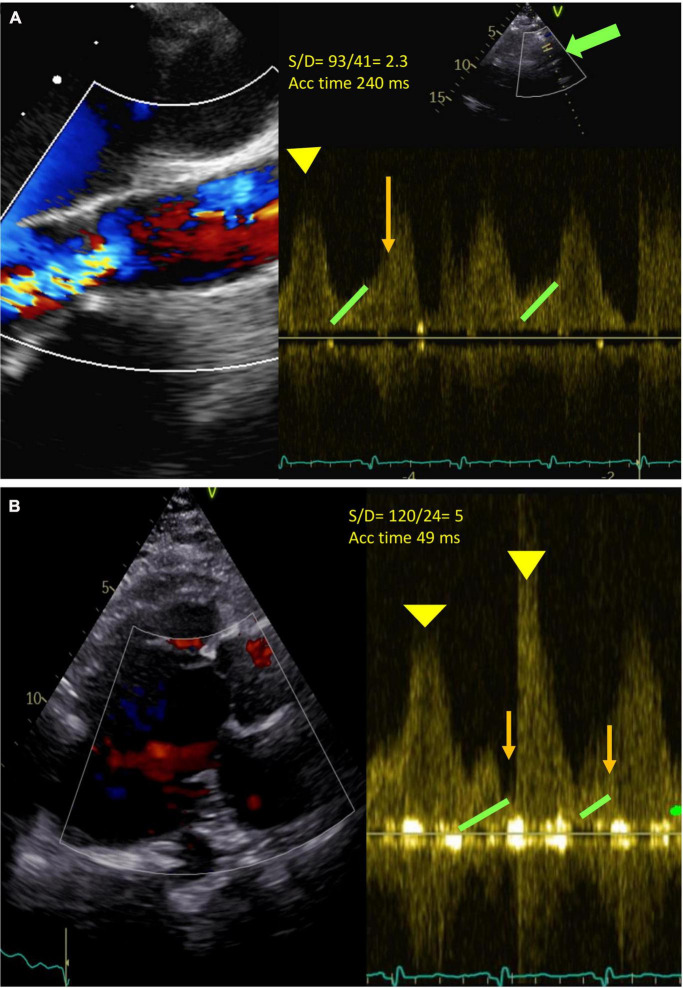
(A) Severe continuous (holo-systolic and holo-diastolic) aortic regurgitation (AR). Green arrow: outflow cannula. Pulse Doppler (PW) at about 1 cm from the outflow cannula (bold green arrow). Diastolic acceleration time 49 ms (orange line), S/D ratio 93/41 = 2.3 (<5 significant AR) (Yellow head arrow: systolic wave; green head arrow: end Diastolic velocity). Acceleration time 240 ms. (B) No AR. S/D 53/10 = 5. Acceleration time 49.
